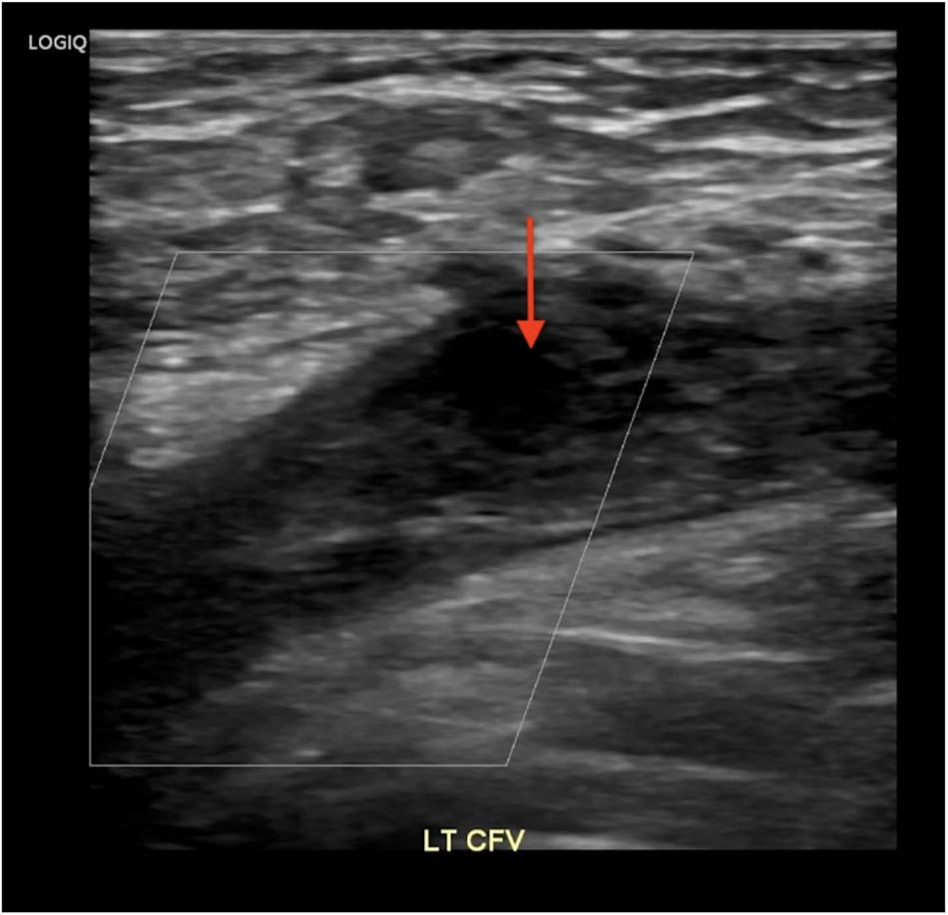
Duplex venous ultrasound of the left lower extremity demonstrating left common femoral vein thrombosis. DVT appears as a hypoechoic area (arrow) indicating turbulent flow in the common femoral vein. Abbreviations: LT—Left, CFV—Common femoral vein.
